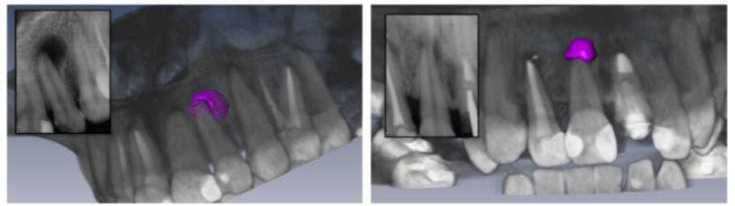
Two examples of periapical lesions reconstructed in 3D using the Amira program, along with the respective radiographic images
